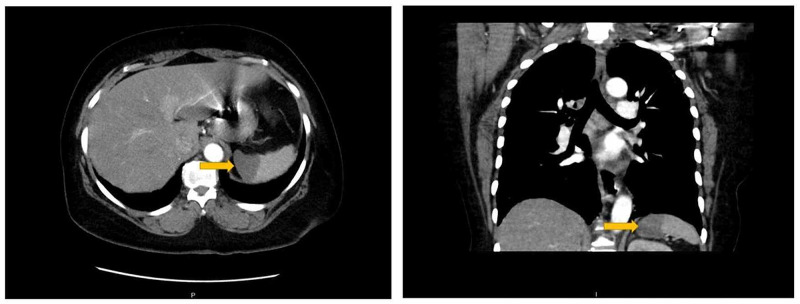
Axial and coronal view of the CT chest-abdomen showing area of low attenuation on the medial side of the spleen
